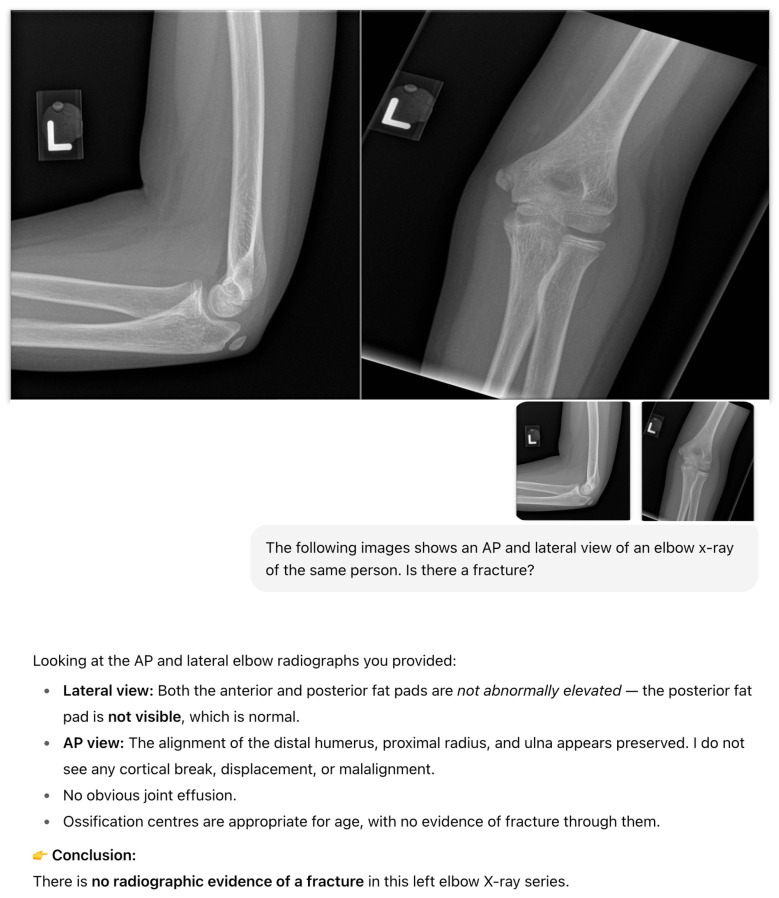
Sample elbow radiographs from an 11-year-old child with no fracture and ChatGPT-4o’s output.
